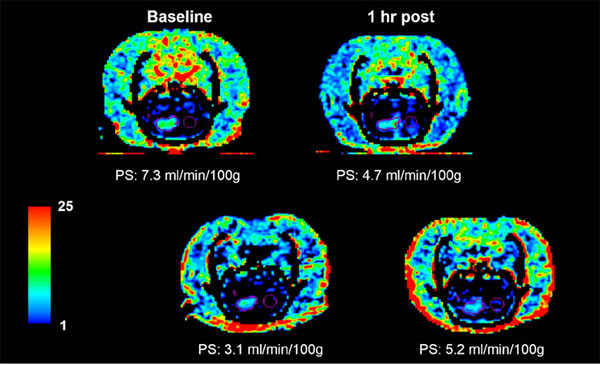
Blood-tumor-barrier (BTB) permeability surface-area product (PS) maps at baseline,1, 24, and 72 h following focused ultrasound (FUS) and microbubble (MB) treatment
